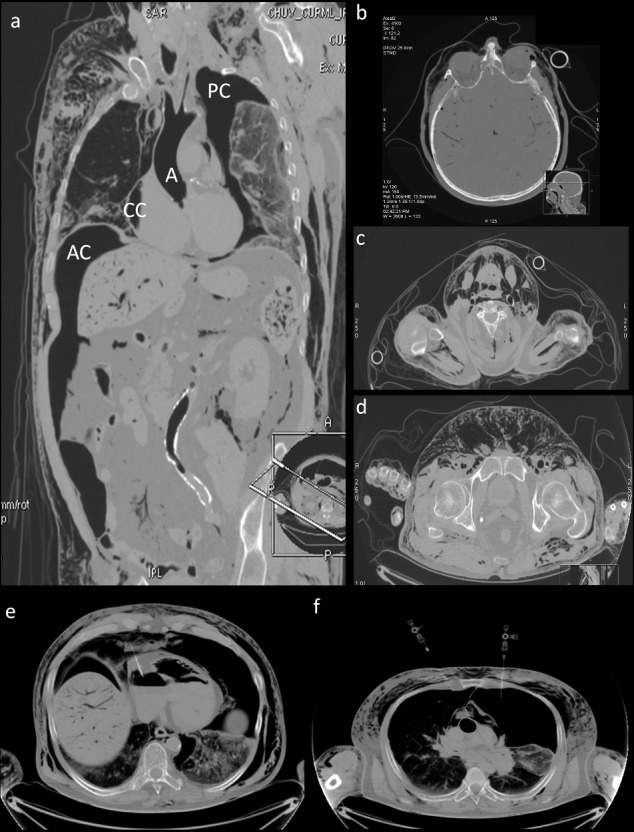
Images obtained by native post-mortem CT showing massive accumulation of gas in all anatomical compartments. (a) Presence of gas in the thorax, including gas in the cardiac cavities (CC), the pleural cavities (PC), the abdominal cavity (AC) and the aorta (A). (b–d) Axial images obtained at the level of the head (b), the neck (c) and the pelvis (d) showing the presence of gas in the soft tissues and the blood vessels of the brain. (e) and (f) Images obtained during gas sampling showing needles in the sampling position in the cardiac cavities (e) and the pleural cavities (f).
